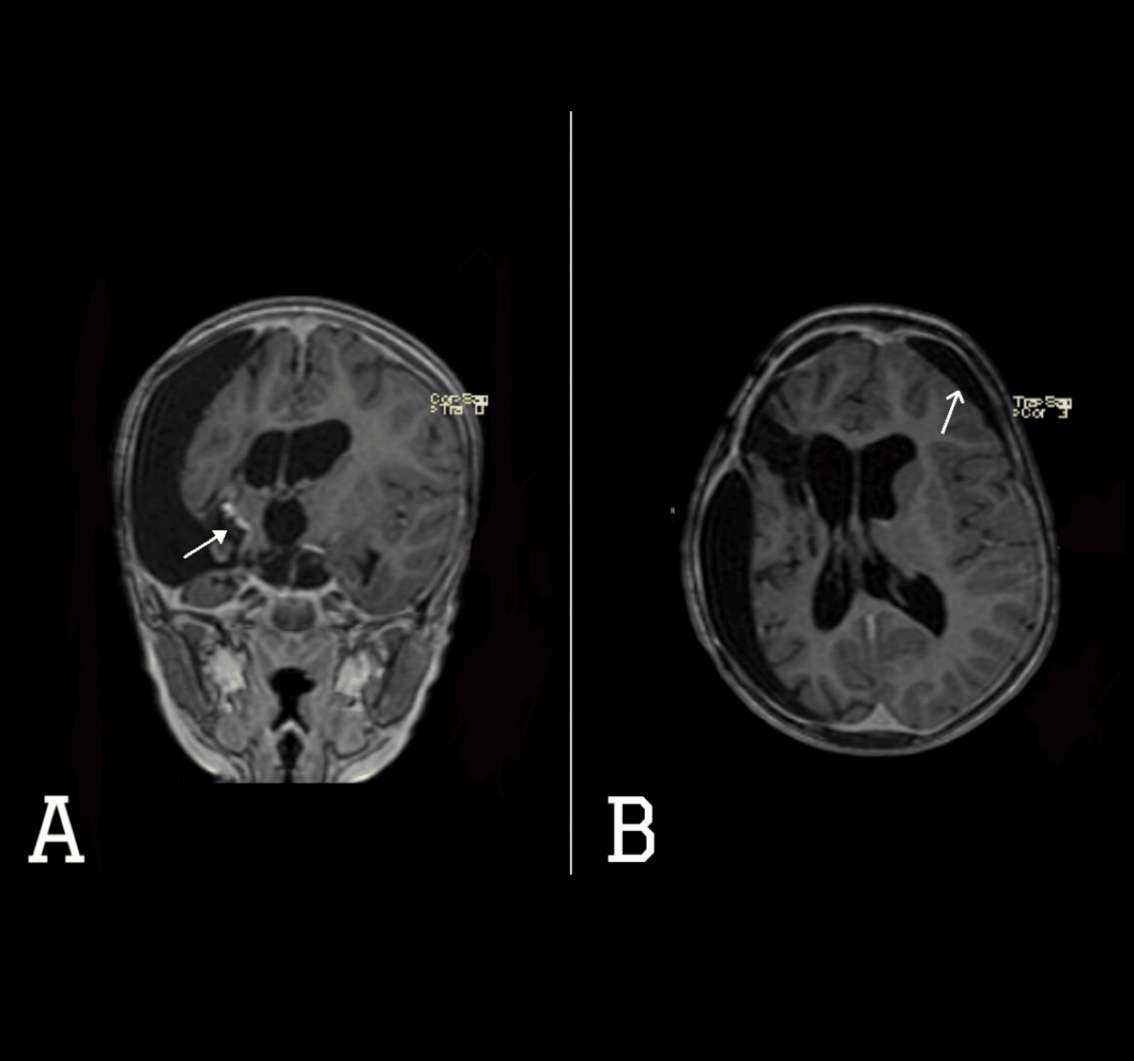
MRI brain with contrast (A) axial and (B) coronal views showing a redemonstration of right frontal parietal craniotomy for resection of the frontotemporal mass with a persistent enhancement of the dural reflections (white filled arrow) more on the right side. Encephalomalacia and gliosis involving the surgical cavity at the right frontal and temporal region marked a decrease in the left subdural fluid collection (White empty arrow) in comparison to the previous study. The prominent ventricular system showed a mild increase in comparison to the previous study likely related to a decrease in the mass effect related to the extra-axial collection.
